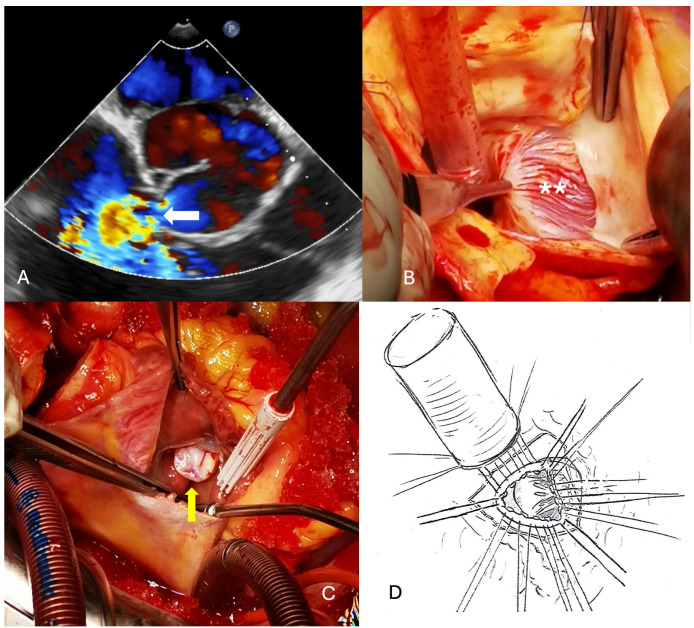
(A) Intraoperative echocardiography showing the ruptured sinus of valsalva aneurysm (white arrow). (B) Exposure of the aortic wall defect (SVA) (white ) below the right coronary ostium that has been detached. (C) Exposure of the defect with the rupture into the right atrium (the aneurysmatic sac is protruding into the right atrium) (yellow arrow). (D) Bentall procedure, the patch to reinforce the aortic anulus and the adjacent sinus can be seen under the sutures on the right.
